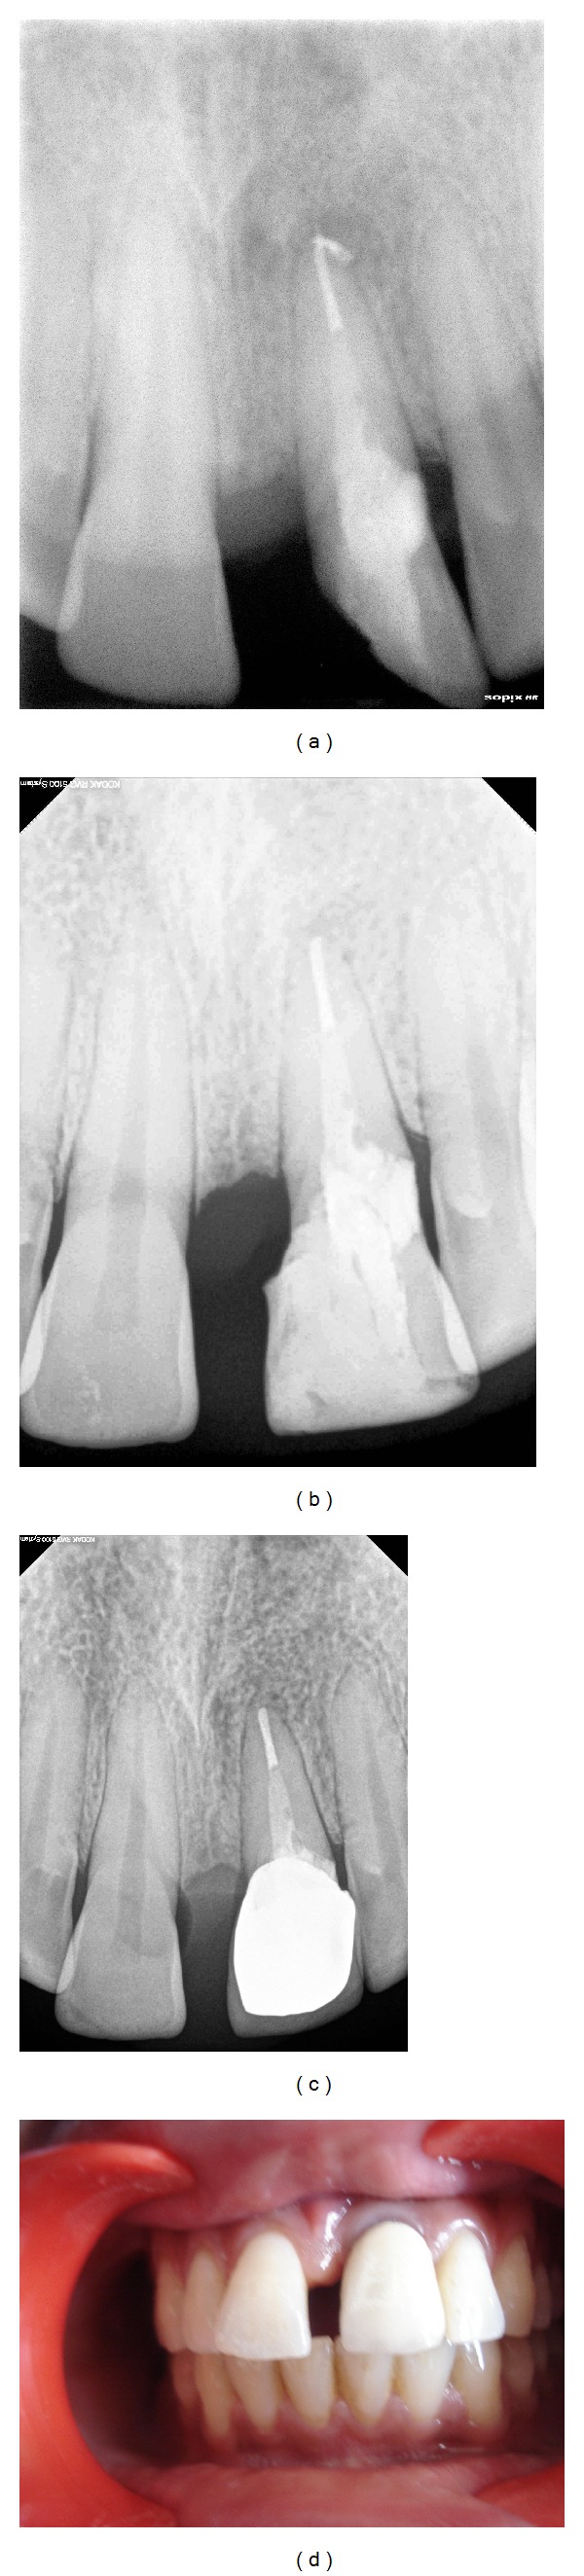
(a) Radiograph showing a complete root canal treatment. (b) 1-year followup showing no sign of Periapical pathosis. (c) 4-year followup radiograph. (d) 4-year followup clinical photograph.
